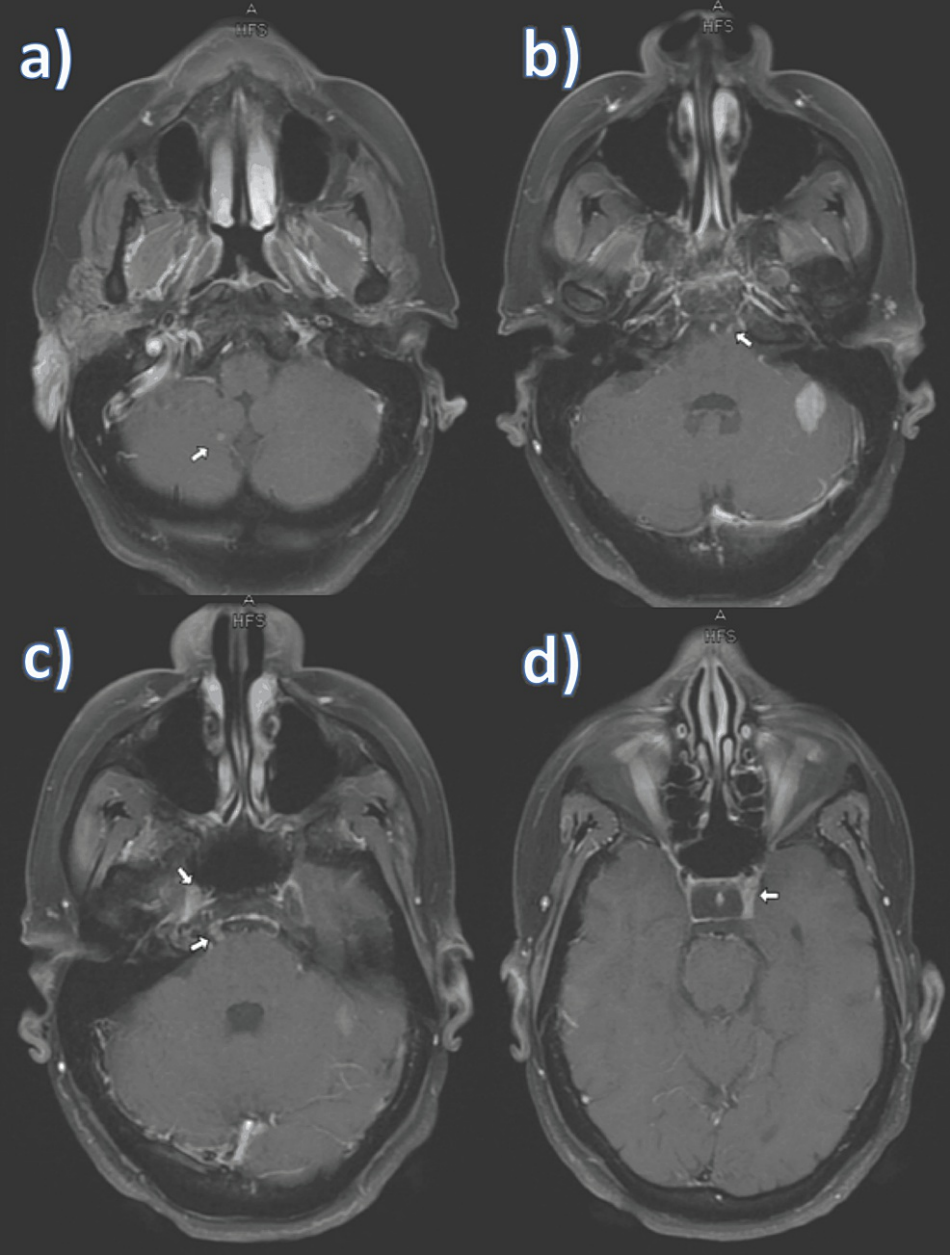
Brain MRI. a) Enhancing cerebellar lesion on the right. b) Abnormally-enhancing cerebellar lesion on the left and left cranial nerve (CN) VI enhancement. c) Enhancement of the right Meckel’s cave (upper arrow) and slight enhancement of the right CN VI. d) Bilateral enhancement of the prepontine cisterns.
